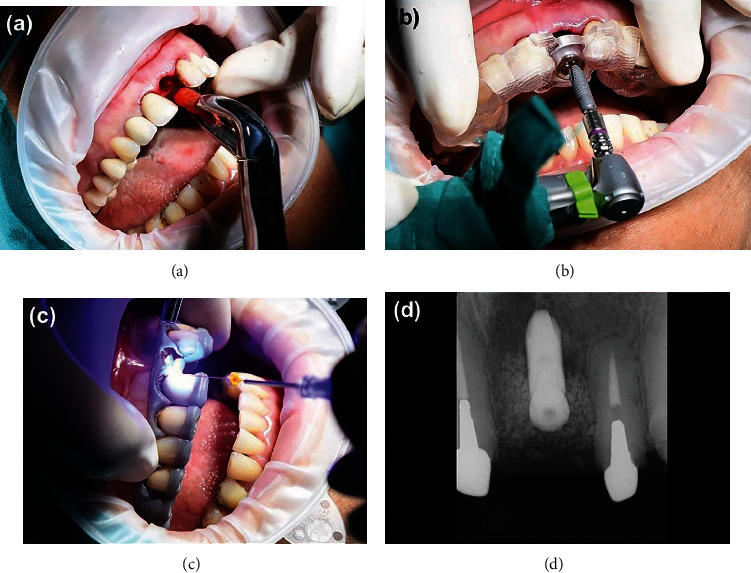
Atraumatic extraction of tooth 21 (a), dental implant placement using a surgical guide (b), crown lengthening by using a laser (SiroLaser Blue Dentsply Sirona, Bensheim, Germany) with digitally assisted crown lengthening guide (c), and periapical radiograph post dental implant placement (d).
